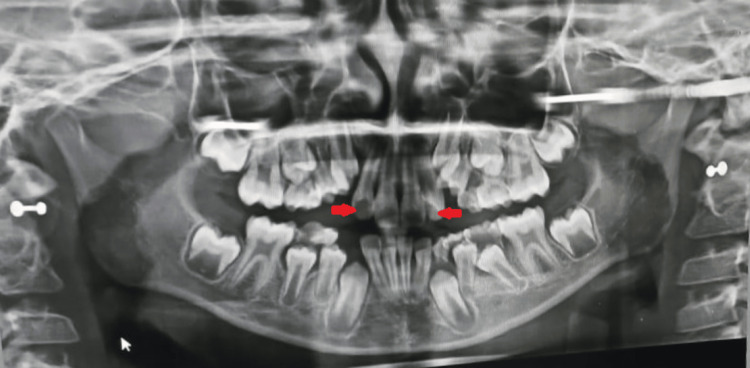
Panoramic radiograph showing upper bilateral dens invaginatus
